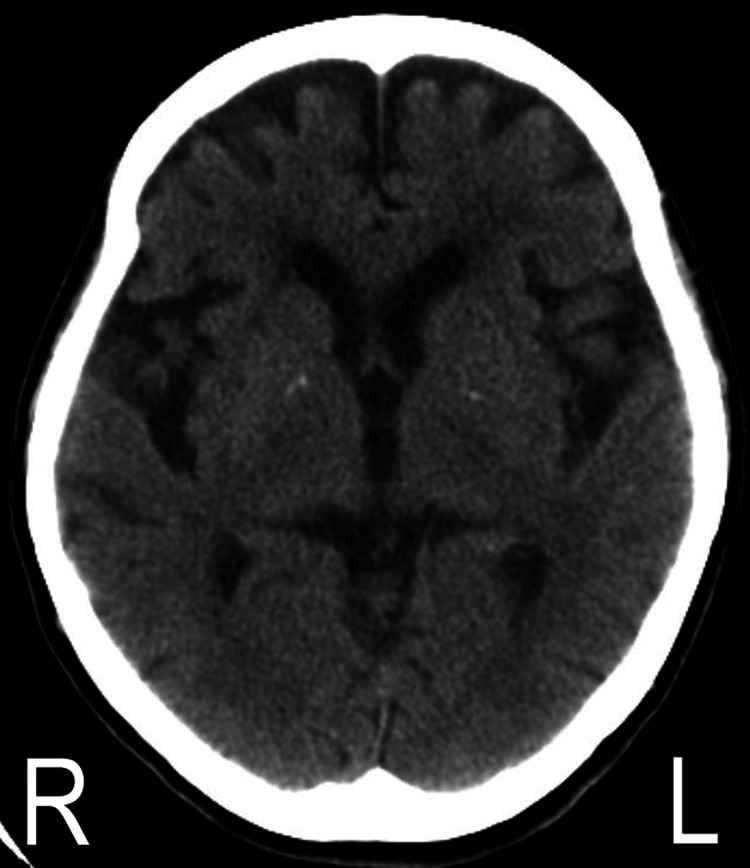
Brain CT scan on admission An axial image at the level of basal ganglia shows bilateral mild frontotemporal atrophy.
